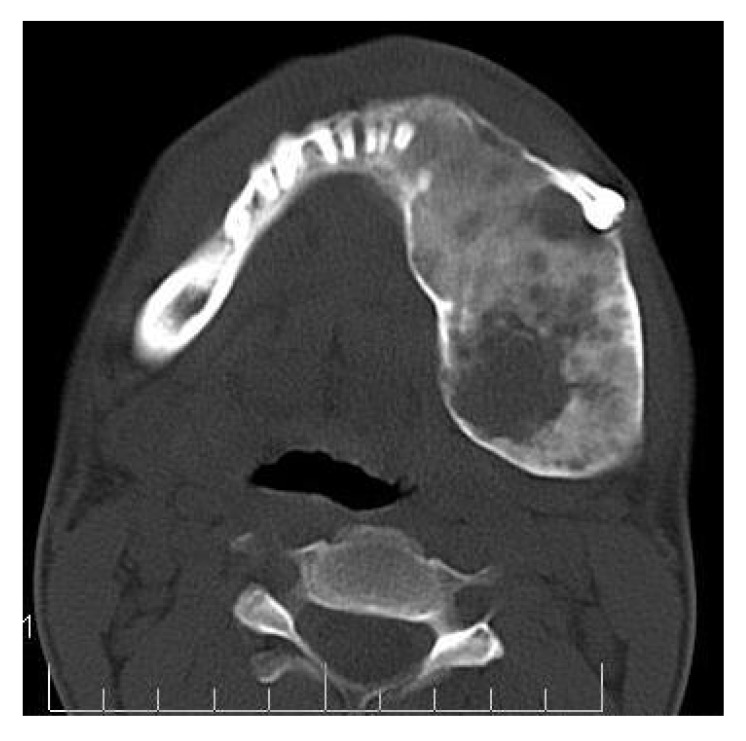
Axial CT section reveals expansion of the mandibular body with erosions and destruction of the internal structures. Tooth displacement was also observed.
